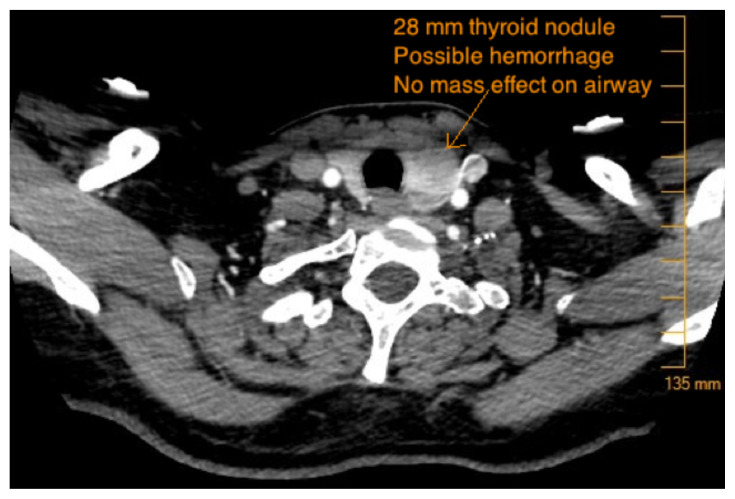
Axial CTA Video Link: https://youtu.be/Hxoxi8FHvmk [Fig f1-jetem-5-3-v10][Fig f2-jetem-5-3-v10][Fig f3-jetem-5-3-v10]Sagital CTA Video Link: https://youtu.be/Xx_HeC6N6Es Coronal CTA Video Link: https://youtu.be/Vuophy3ublE Axial CT Soft Tissue Video Link: https://youtu.be/K1mMRIlBVqg
